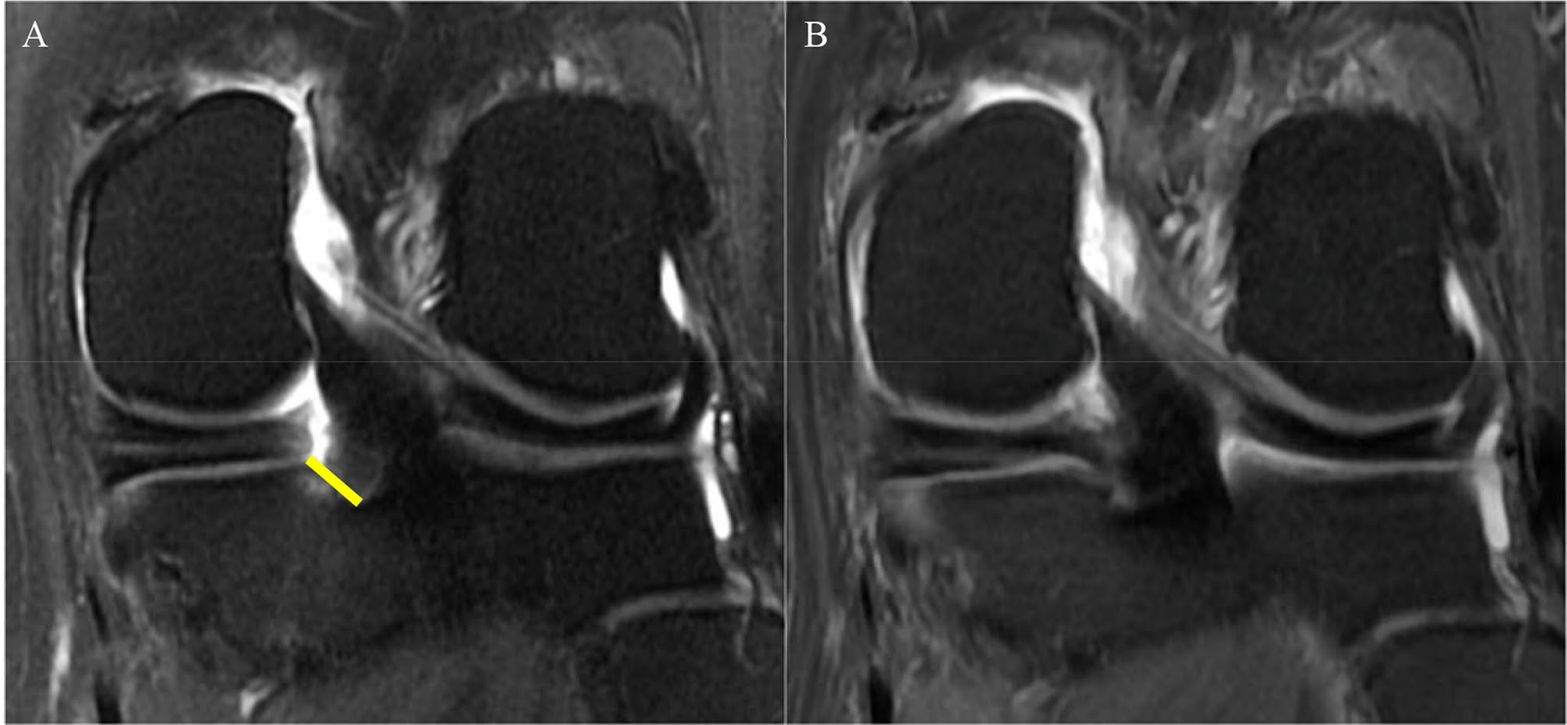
A 58-year-old woman presented with right knee pain and swelling for a month. (A) Baseline MRI showed a radial tear at the posterior root of the medial meniscus. The distance from the torn meniscal root to its bony attachment was 8 mm (yellow line). Interestingly no significant meniscal extrusion was noted at this time. (B) At 7 months after the arthroscopic meniscal root repair. The meniscal root showed complete healing. Note the slightly increased signal intensity in the repaired meniscal root, which is a normal post-operative finding [17]
